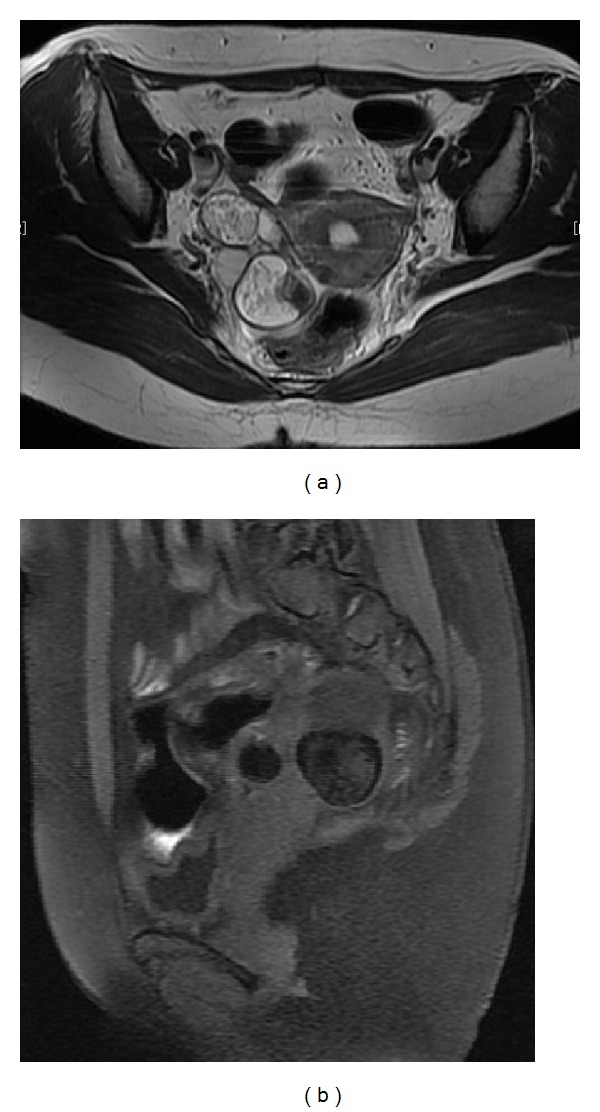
Right ovarian cyst with fat in cystic cavity before second surgery. (a) Axial T2-weighted image shows high-signal intensity cyst. (b) Fat-saturated T1-weighted image shows signal decrease on the cyst.
